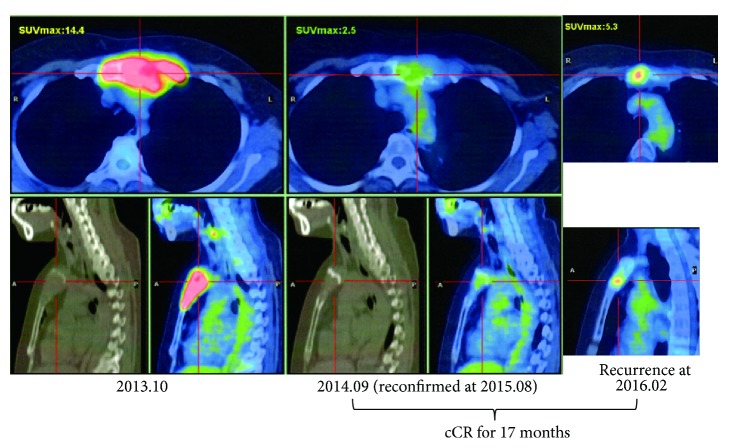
PET-CT of the sternum of Case #20 (assigned as cCR Case 3). A huge bone metastasis of breast carcinoma was observed. The length of cCR status was calculated between the reconfirmation of disease-free status on September 3, 2014, and the diagnosis of local recurrence on February 9, 2016.
